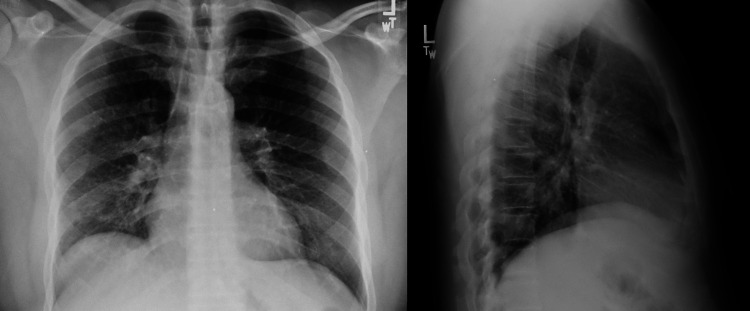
The chest X-ray showed mild hazy opacity in the right lower lobe, worrisome for early or developing pneumonia.
